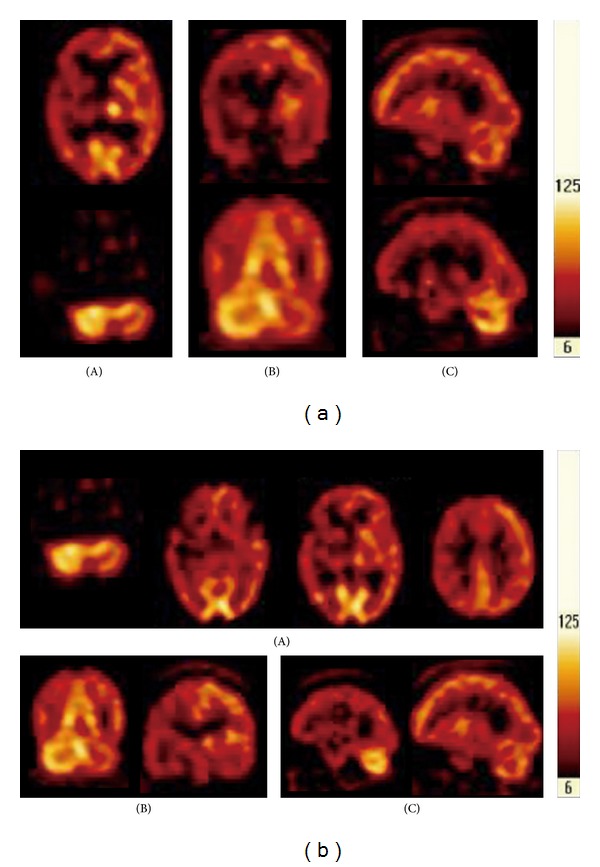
(a) The pictures of the brain SPECT. (A) Transverse view; (B) coronal view; (C) sagittal view. There is inhomogeneous perfusion in the cerebral cortex with relative decrease of uptake in right fronto-temporal-parietal, right temporal, and right parietal regions. The uptake in right basal ganglion and thalamus is decreased when compared with the left side. Remarkably, the uptake in left cerebellum looks lower than the right cerebellum, suggesting the presence of CCD. (b) The second examination of the brain SPECT. (A) Transverse view; (B) coronal view; (C) sagittal view. The pictures checked in the second time are similar to the first, and interestingly enough, CCD remains in the left cerebellum.
